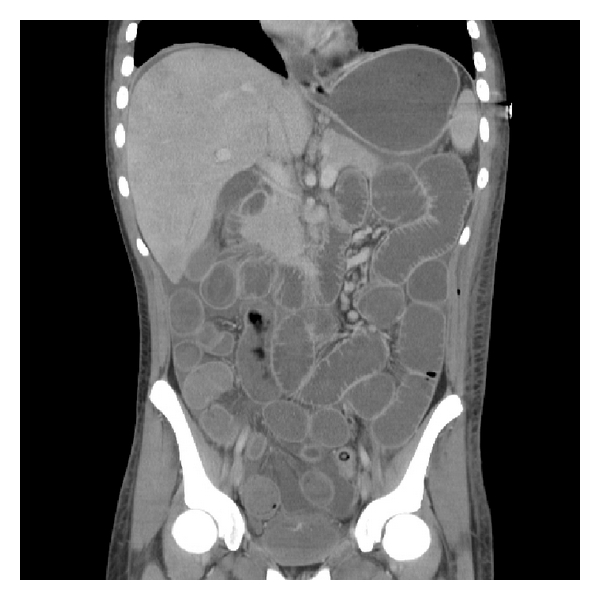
Abdominal CT showing multiple oedematous and dilated loops of both small and large bowel.
